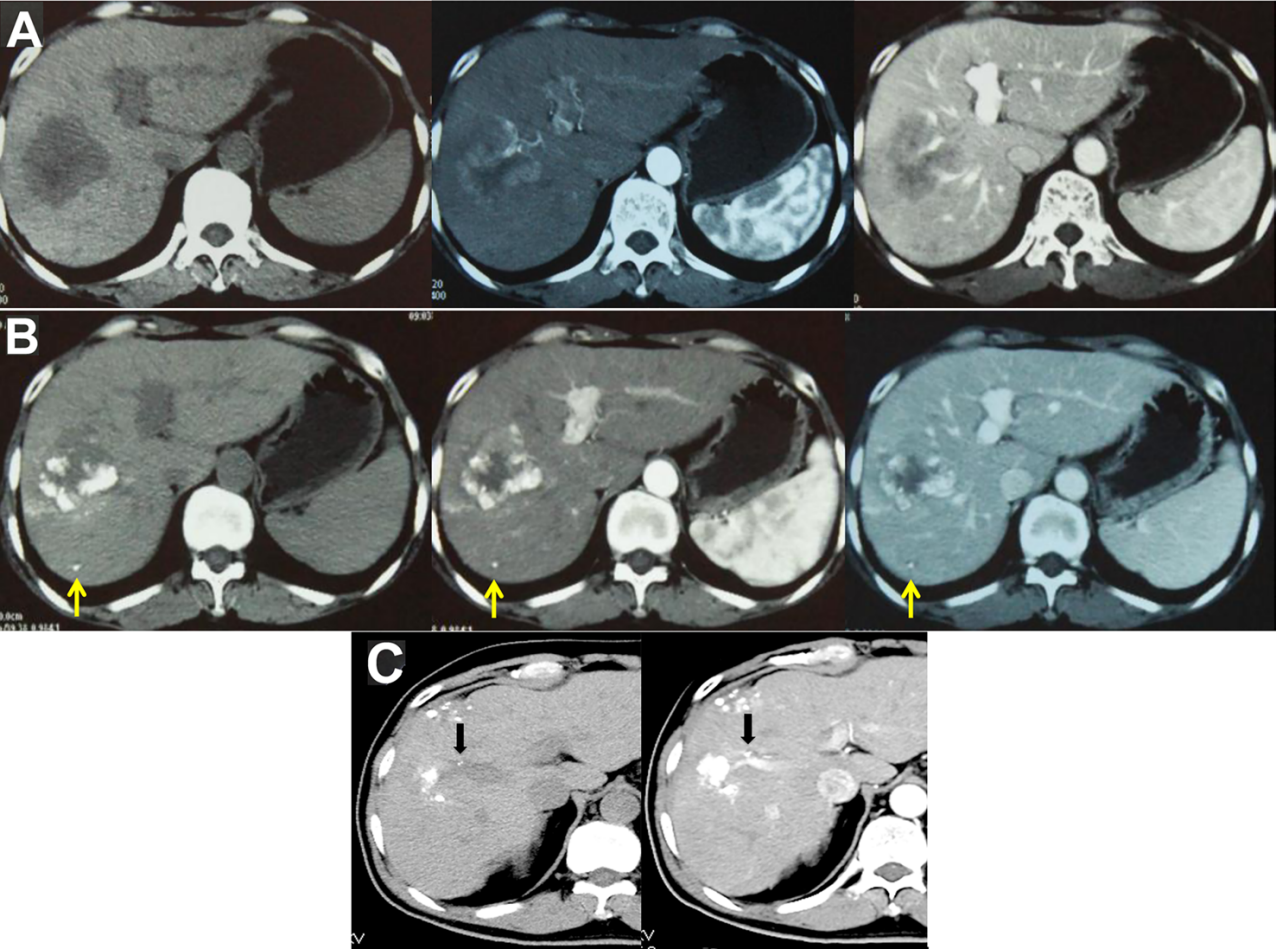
TACE-CT enables detection of focal loci and precise staging. (A) CT before treatment showed a 7-cm tumor in the S5 and S6 region of the liver; (B) Follow-up CT examination at 4 weeks after TACE showed that the tumor volume at S5 and S6 was reduced, while a small lesion was detected at S6, as indicated by the arrow; (C) In another male patient, follow-up CT examination at 3 weeks after TACE showed intravascular lipiodol deposits (indicated by the arrow), suggesting the formation of an intravascular small tumor thrombus.
